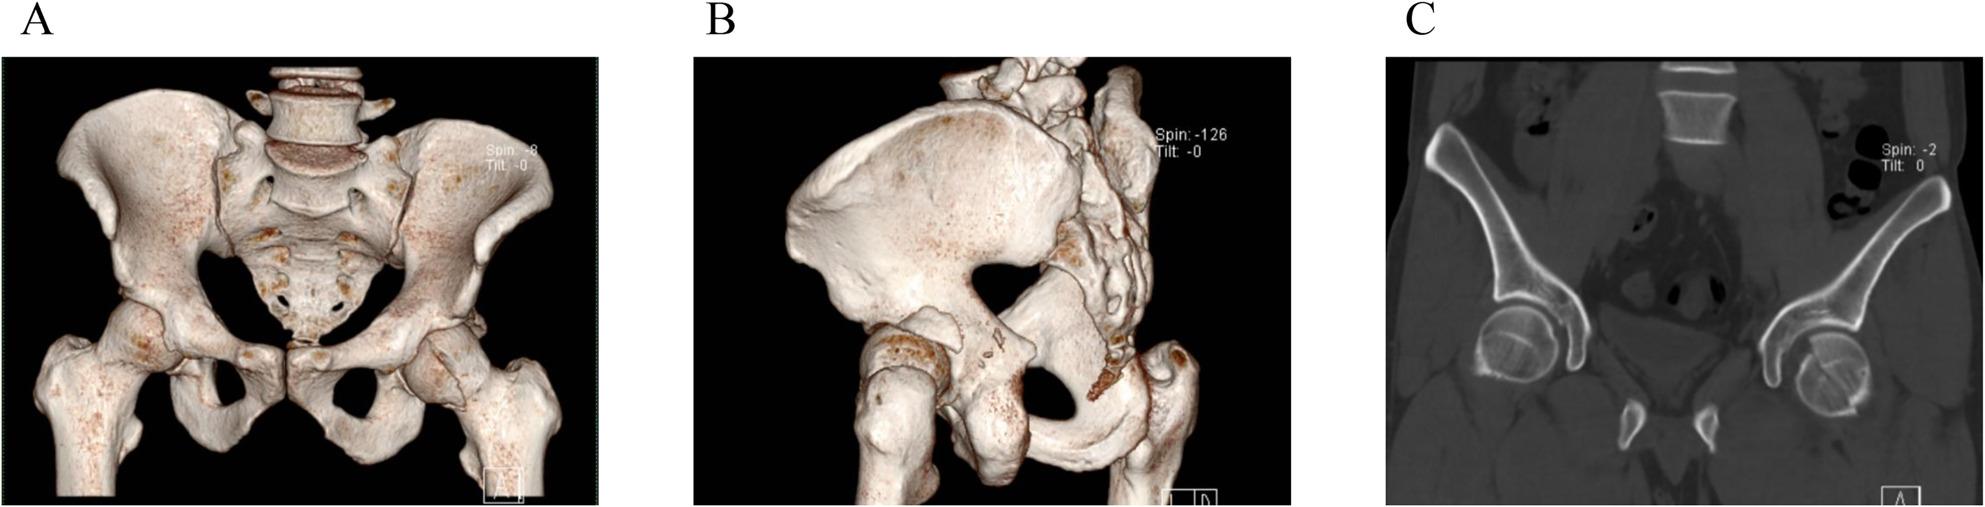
A 32-year-old male patient with a left hip injury caused by a traffic injury. Diagnosis: Fracture of the left femoral head (Pipkin type IV), fracture of the left posterior wall of the acetabulum. Greater trochanter osteotomy was performed 7 days after injury using Ganz approach under general anesthesia. The left acetabular fracture and left femoral head fracture were treated with open reduction and internal fixation. The acetabular fracture was fixed with plates and screws, the femoral head fracture was fixed with counterburied screws, and the greater trochanter was fixed with screws and plates. A-C: Preoperative CT scan showed a fracture of the left femoral head (Pipkin type IV) with significant displacement of the fracture end and a fracture of the posterior lateral wall of the acetabulum
